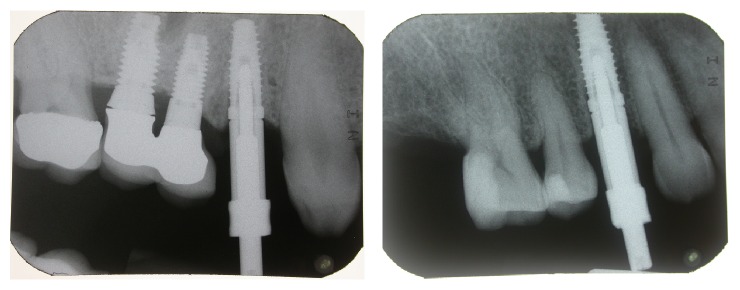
Case 1 and Case 2: after implant placement and connection of the impression transfer periapical radiograph.
